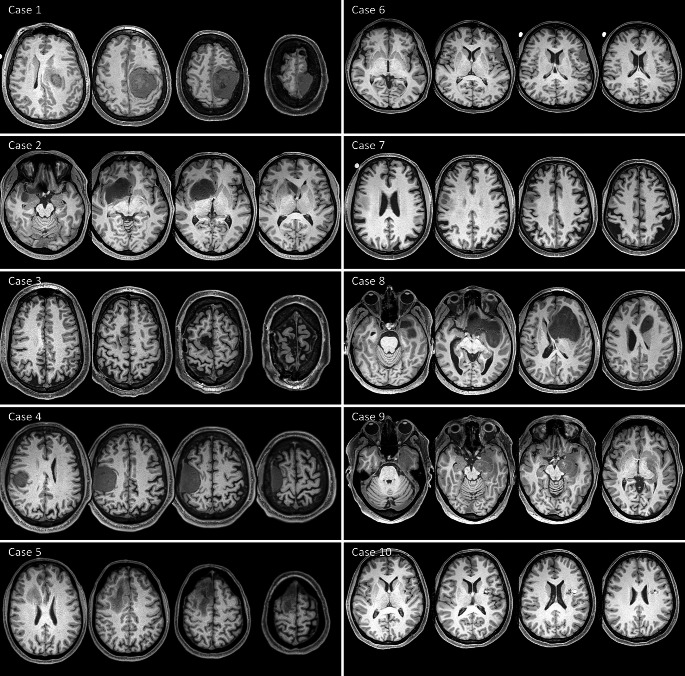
Tumor distribution at baseline. Axial slices of the structural MRI for each subject (native space) depicting tumor location. The first five cases (left side) are ‘motor patients’, the second five cases (right side) are ‘cognitive patients’
